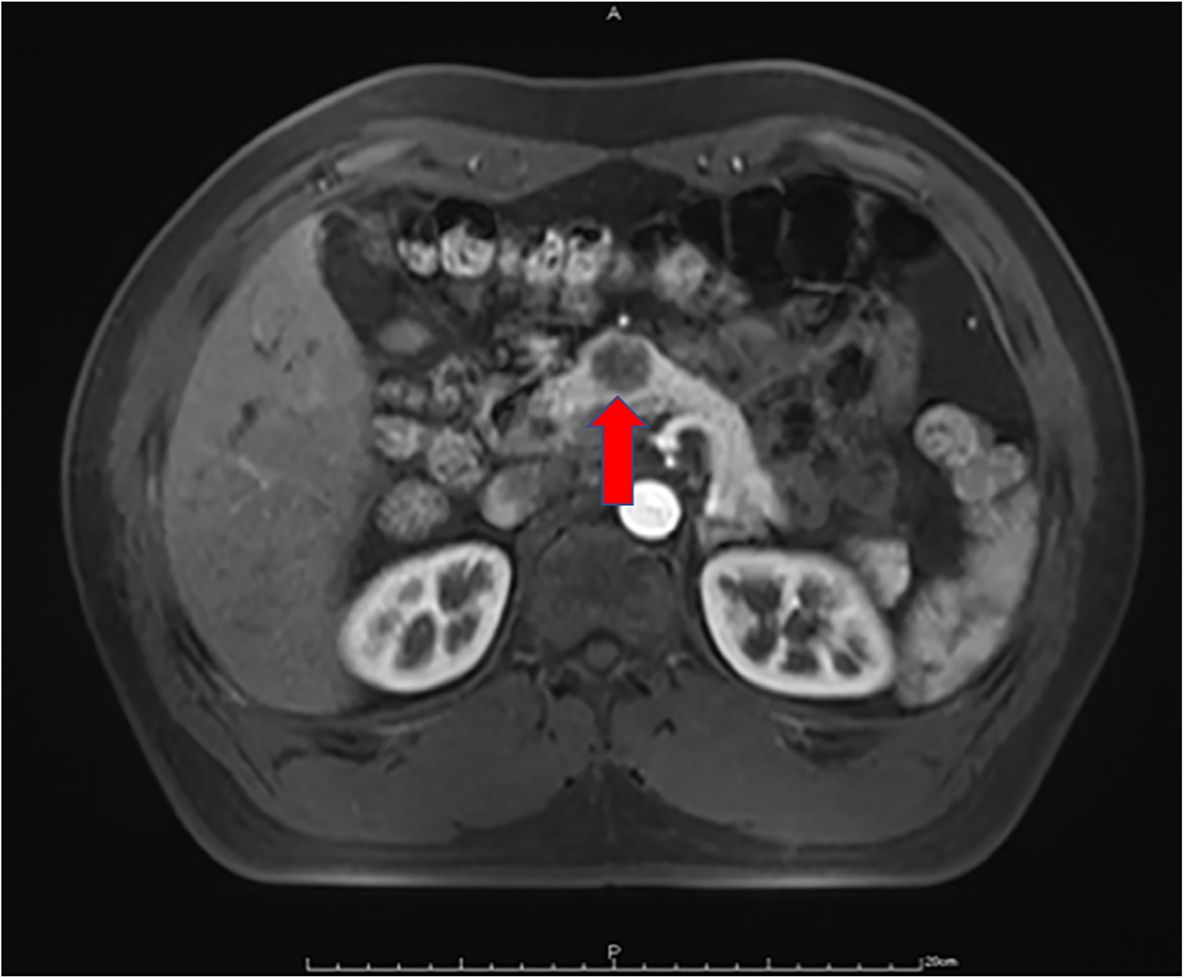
Preoperative MRI showed a tumor in the pancreatic neck (red solid arrow)
